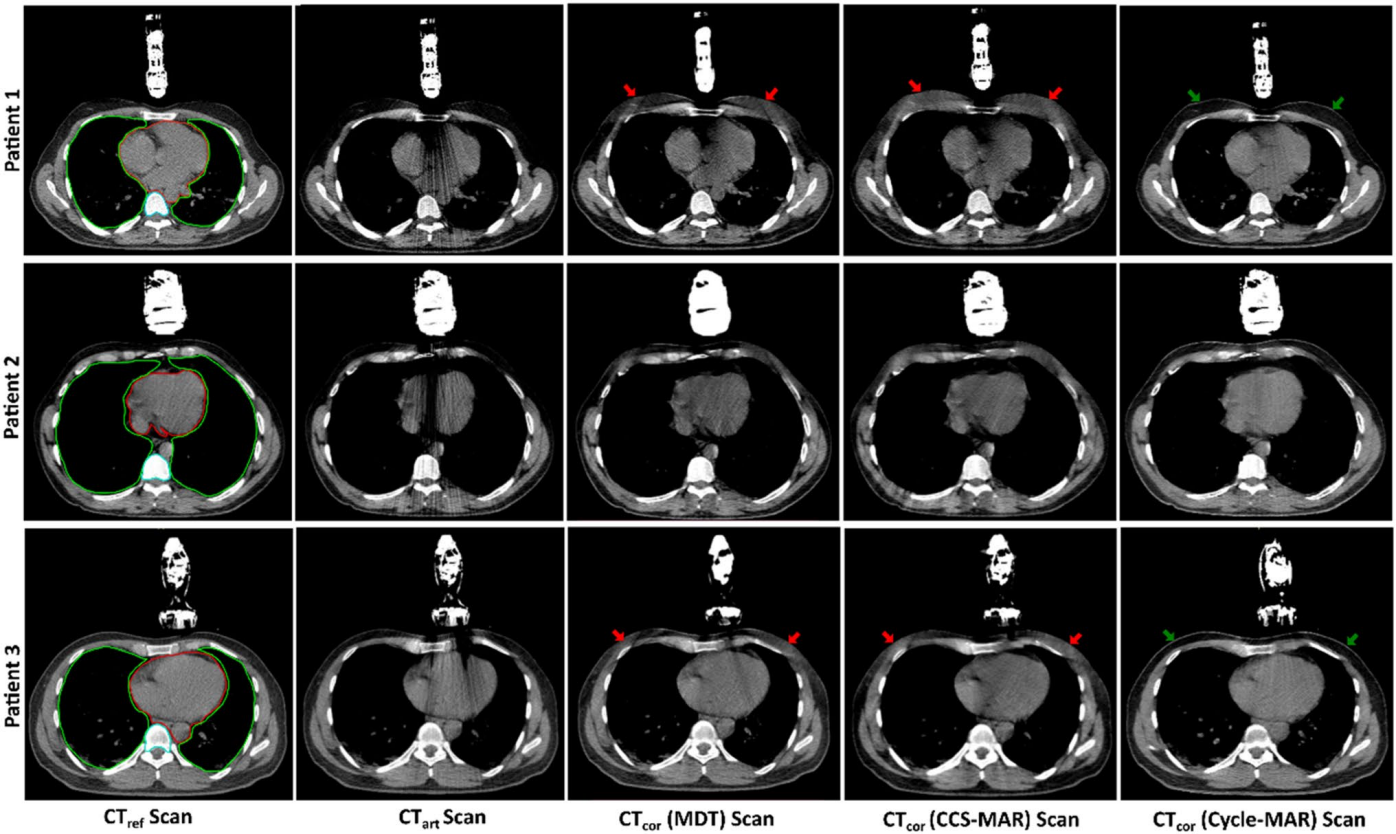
CT scans from three patients. The images from left to right show: the CT_ref_ scan with the US transducer details, CT_art_ scan, and the CT_cor_ scans after MDT, CCS-MAR, and Cycle-MAR applications [Window level/width: 50/350]. The red and green arrows indicate the changes of soft tissue density. The counters for the HU value measurements were shown on the CT_ref_ scans: the heart (red), bone (blue) and lungs (green)
